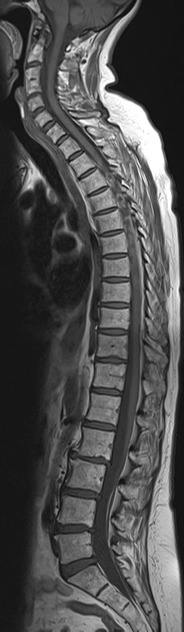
Sagittal T1-weighted MRI of the whole spine in a 63-year-old female patient with back pain. Block vertebra formation C3/4. The number of mobile vertebrae is reduced by one. Assuming seven cervical and 12 thoracic vertebrae, there are four morphological lumbar vertebrae, and there is no transitional vertebra as such
